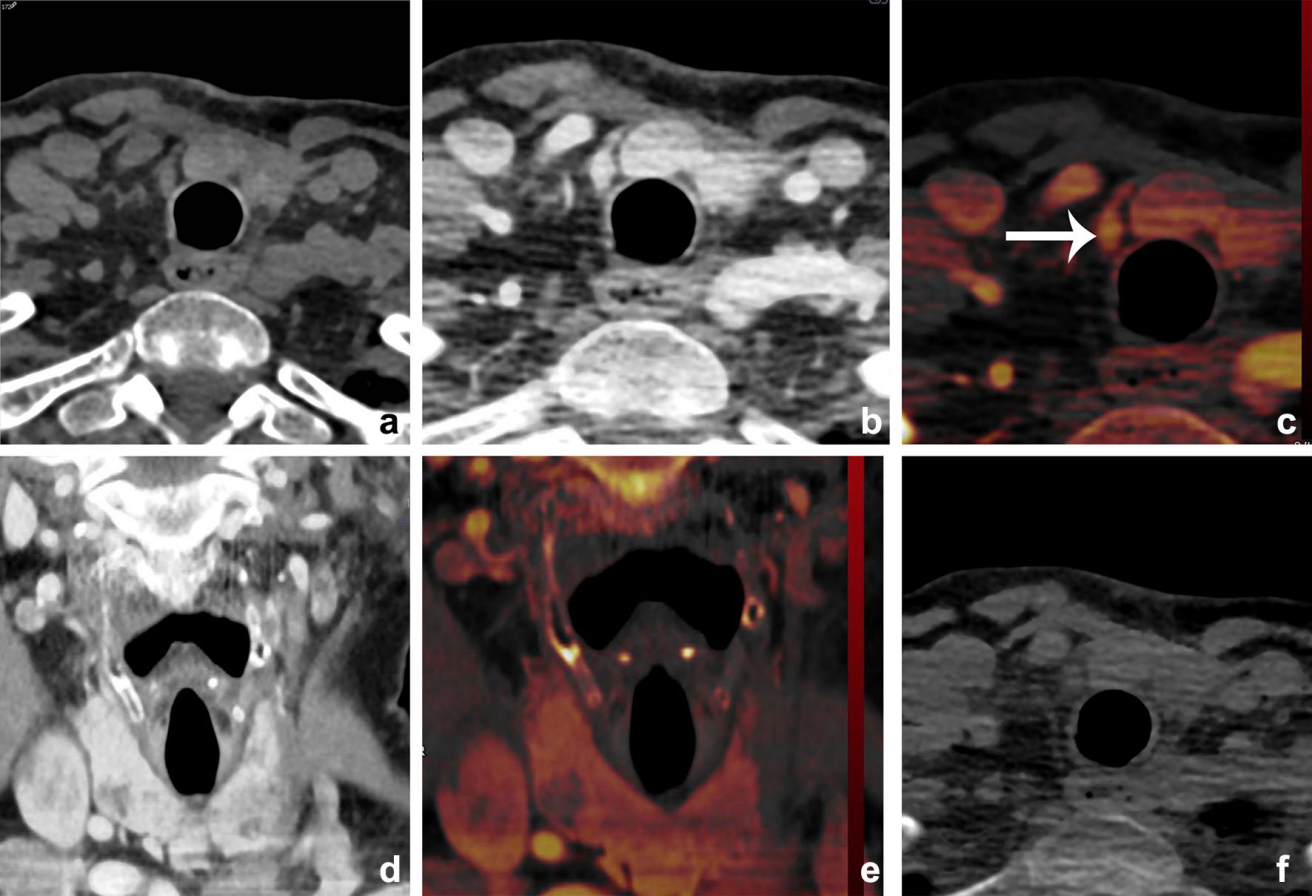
A 63-year-old female presented with primary hyperparathyroidism. Initial Choline PET imaging and MIBI scanning were negative. A multiphase CT showed a small enhancing lesion just caudal to the right thyroid lobe, which was proven a parathyroid adenoma (*arrow*). Axial noncontrast images (a), 30-sec axial-mixed images (b), iodine fusion images (c), and coronal mixed (d), and fusion (e) images. A Virtual noncontrast image is shown at (f), the latter demonstrating more noise and streak artifacts than the true nonenhanced scan, although still diagnostic. Noncontrast CT: 80 kVp 277 mAs; CTDI 5.34 mGy; DLP 113.4 mGycm. DECT 30 s: 80/150 kVP 61/31 mAs; CTDI 5.52 mGy; DLP 113.0 mGycm
